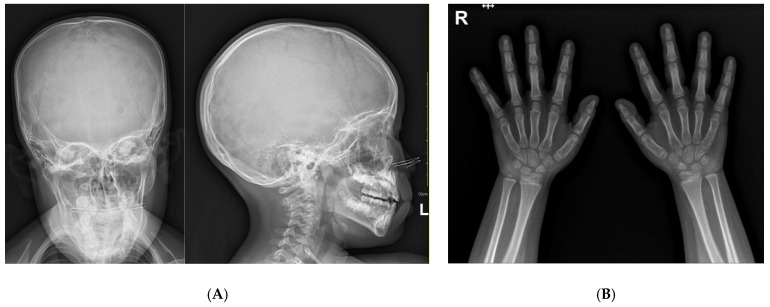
Plain radiography (A) Anteroposterior and lateral of skull series. (B) Both hand X-rays at chronological age of 4 years and 7 months.
